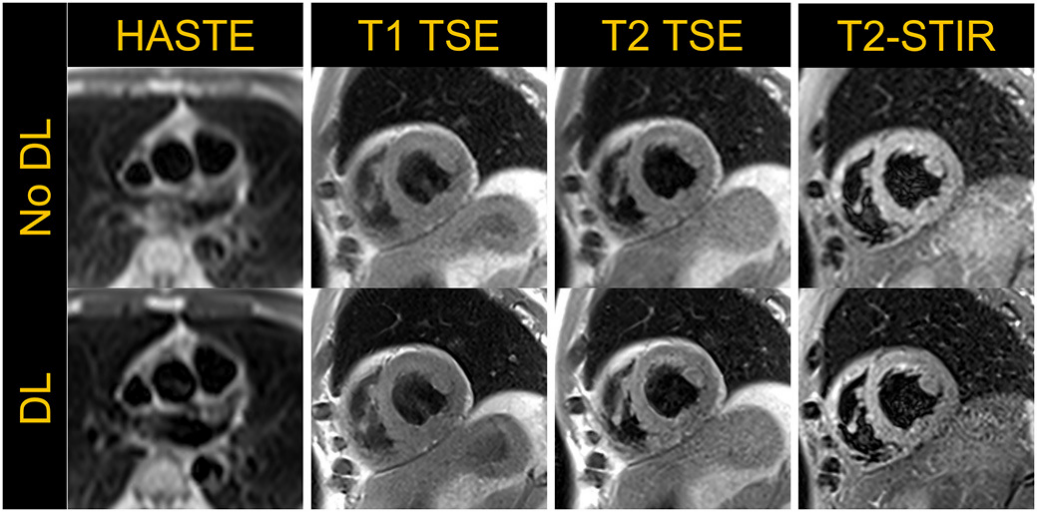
Half-Fourier Acquisition Single-Shot Turbo Spin Echo (HASTE) in an axial view and T1-weighted, T2-weighted TSE and T2-short tau inversion recovery (STIR) images acquired in a mid-short axis view in a volunteer demonstrate the application of dark-blood turbo spin echo based cardiac imaging techniques. The top row shows images without deep-learning (DL) based image enhancement while the bottom row depicts images with increased sharpness resulting from DL-based image reconstruction.
